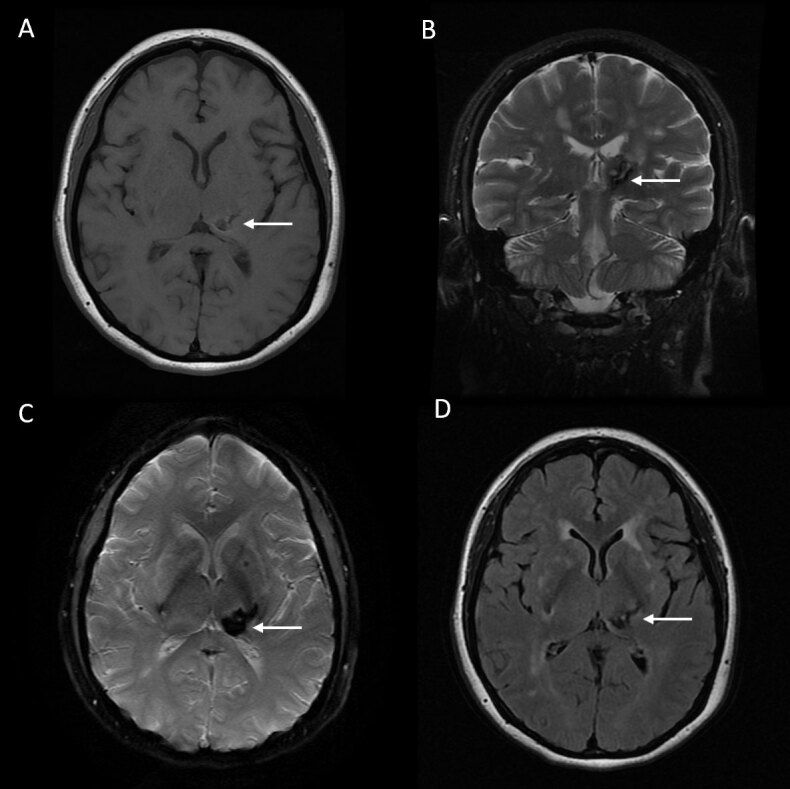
Axial brain magnetic resonance imaging (MRI) T1 (A), coronal T2 (B), axial susceptibility-weighted imaging (SWI) (C) and axial fluid-attenuated inversion recovery (FLAIR) (D) sequences at the time of the patient’s second stroke, showing acute intracerebral hemorrhage in the left thalamus (white arrow), accompanied by smaller foci of microhemorrhages in left globus pallidus and right inferior putamen. Multiple signal changes in the external capsule, periventricular areas, and centrum semiovale are also observed.
